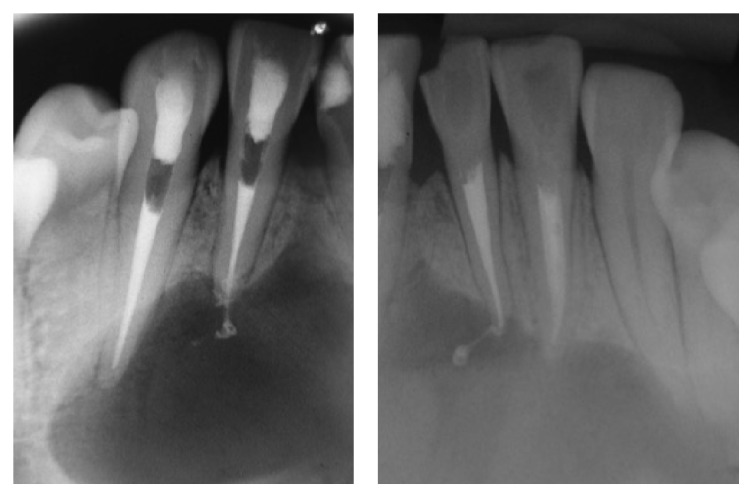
Periapical radiographs obtained immediately after obturation of root canals.
